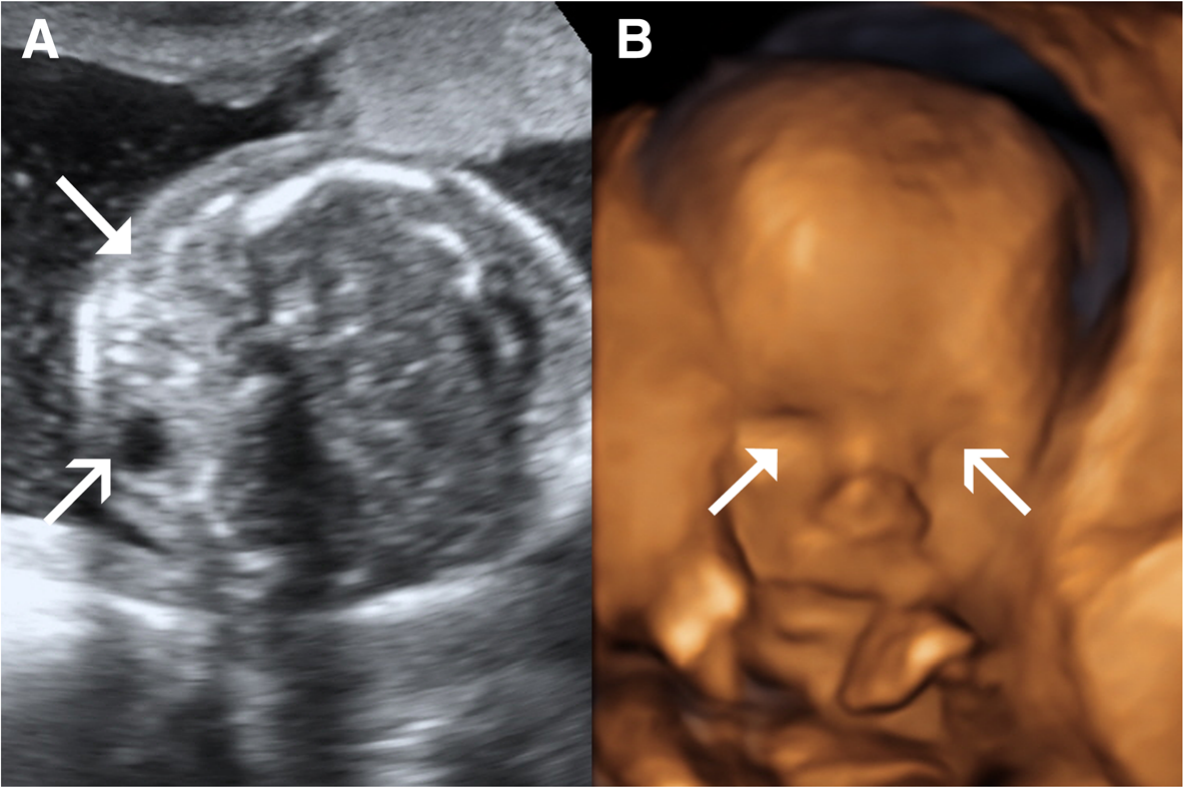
Case B. a Two-dimensional ultrasound findings of anophthalmia. b Three-dimensional ultrasound view of the face showing unilateral anophthalmia. The *triangular arrow-head* represents anophthalmia. The *simple wide arrow-head* represents the normally formed eye
